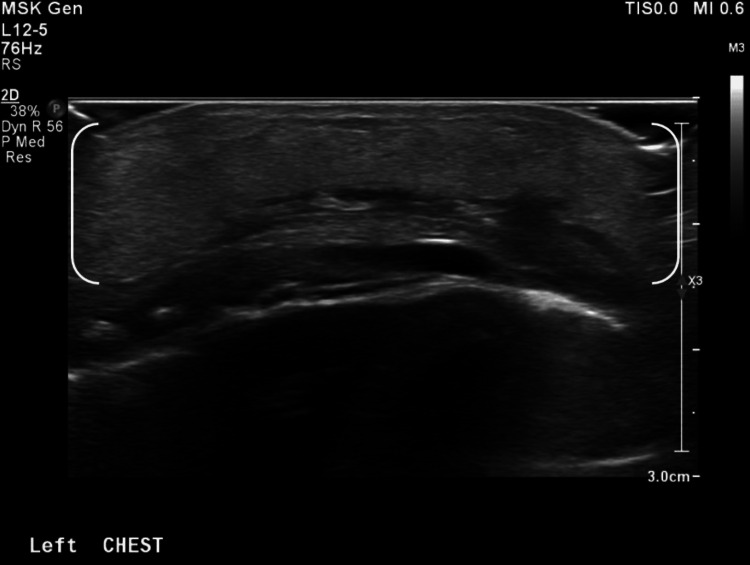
Ultrasound of the left chest wall demonstrating diffuse echogenic widening of the subcutaneous tissues with mild hyperemia
